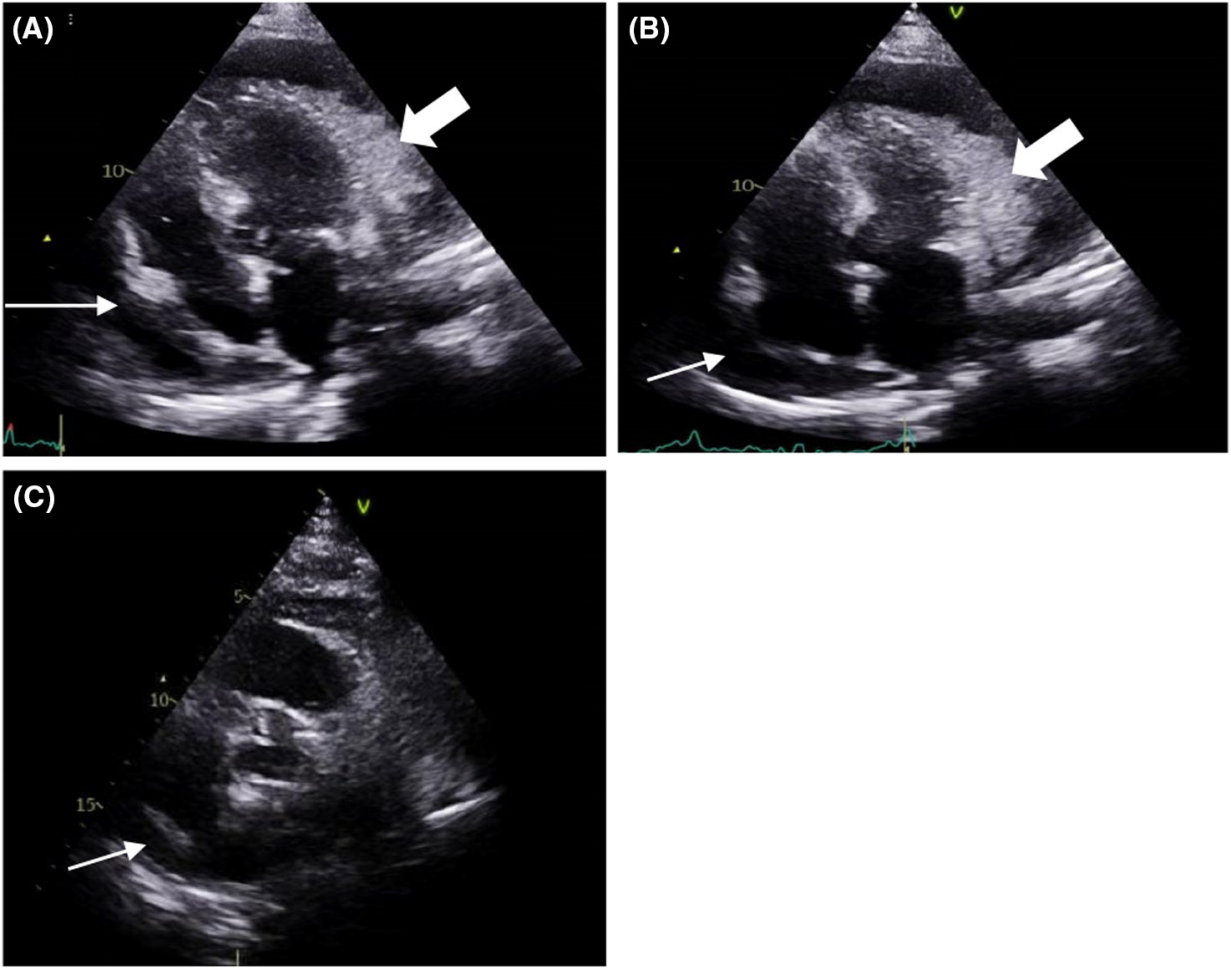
(A and B) Echocardiography upon presentation: showing a large pericardial effusion possibly fibrinous (thin white arrow) with respiratory variation less than 25%. It also revealed an echo‐dense structure seen attached to the visceral pericardium (thick white arrow). (C) Echocardiography post pericardiocentesis and after being started on antituberculous therapy: showing minimal pericardial effusion (thin white arrows).
